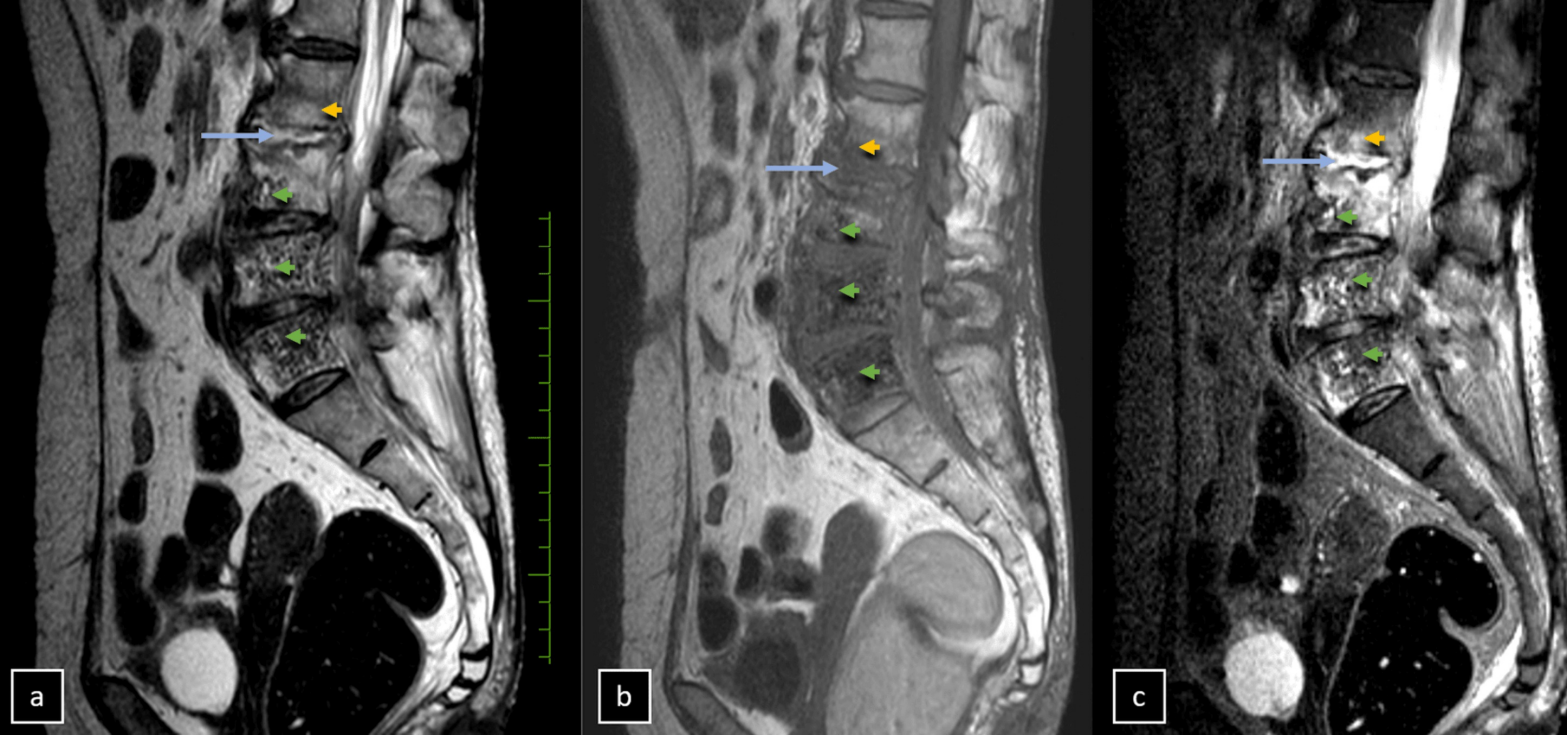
Magnetic resonance imaging (MRI). Sagittal sections (a, b, and c) of T1, T2, and short tau inversion recovery (STIR) sequences depict altered signal intensities in the body of L3, L4, and L5 vertebrae (green arrowheads), suggesting the presence of intra-vertebral air. On T1-weighted images, there is a low signal in the disc space (blue arrow) indicative of fluid, and in adjacent endplates (orange arrowheads), suggesting bone marrow edema. Conversely, T2-weighted and STIR sequences show high signals in the disc space (blue arrow), reflective of fluid accumulation, and in adjacent endplates (orange arrowheads), further indicating bone marrow edema.
